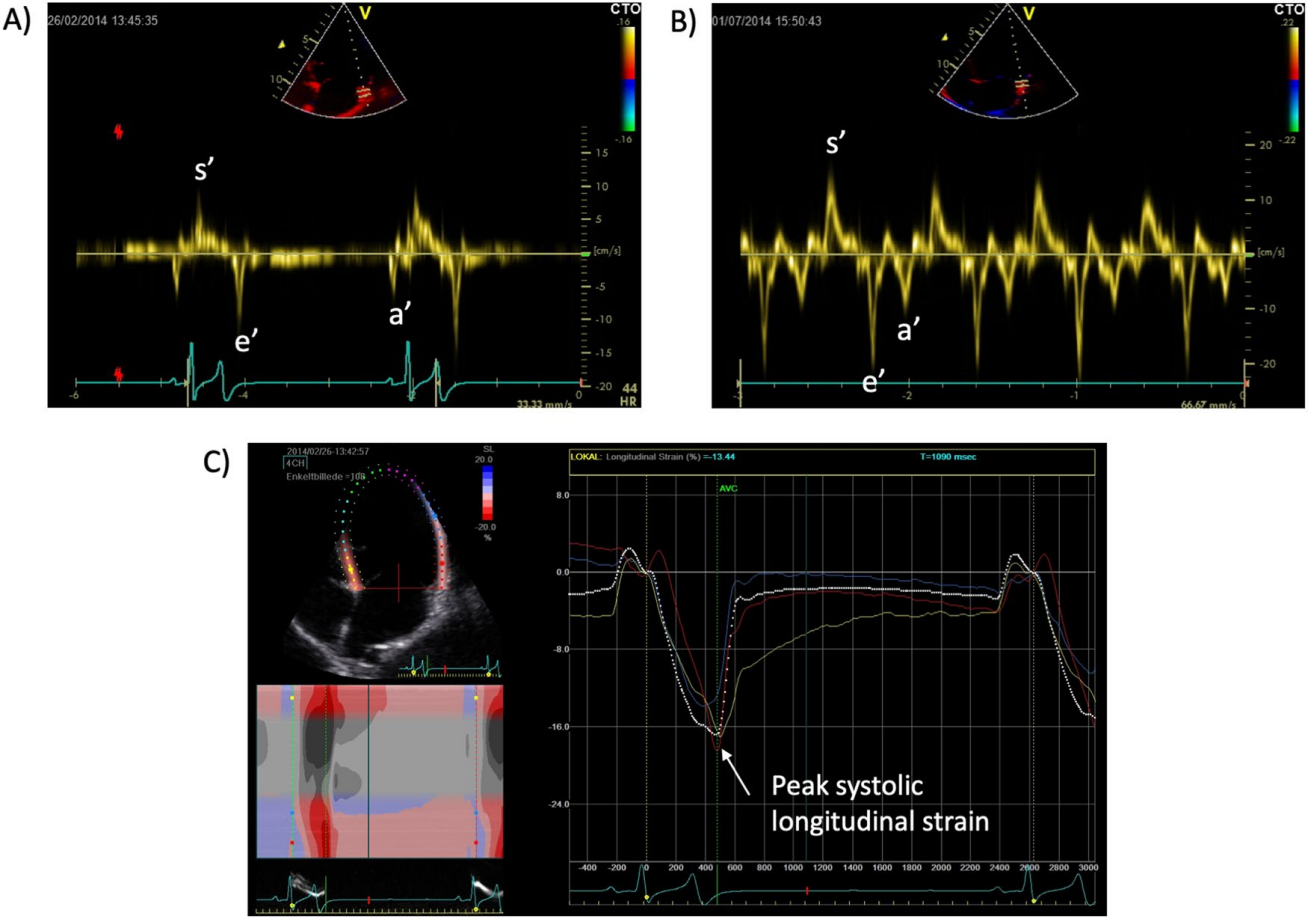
Examples of pulsed wave tissue Doppler echocardiographic and 2D-speckle tracking measurements. S’, e’ and a’ during hibernation (A) and active state (B) and of 2D-speckle tracking strain measurements with an arrow pointing at peak systolic strain (C). Images were exported from EchoPAC 113.1.5.6, GE Vingmed Ultrasound AS, Strandpromenaden 45, 3191 Horten, Norway. URL: https://www.gehealthcare.com/.
